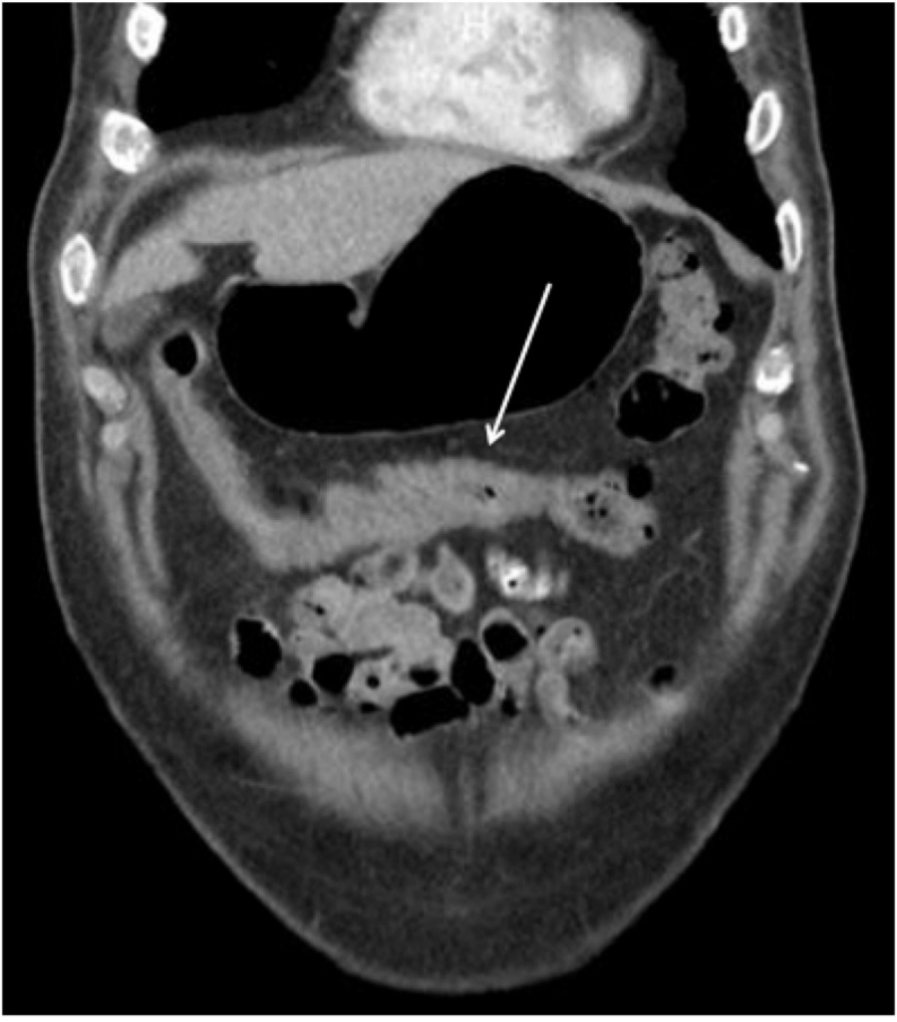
Computed tomography (CT) of abdomen in coronal view demonstrating a large, irregularly shaped lesion of the transverse colon.
